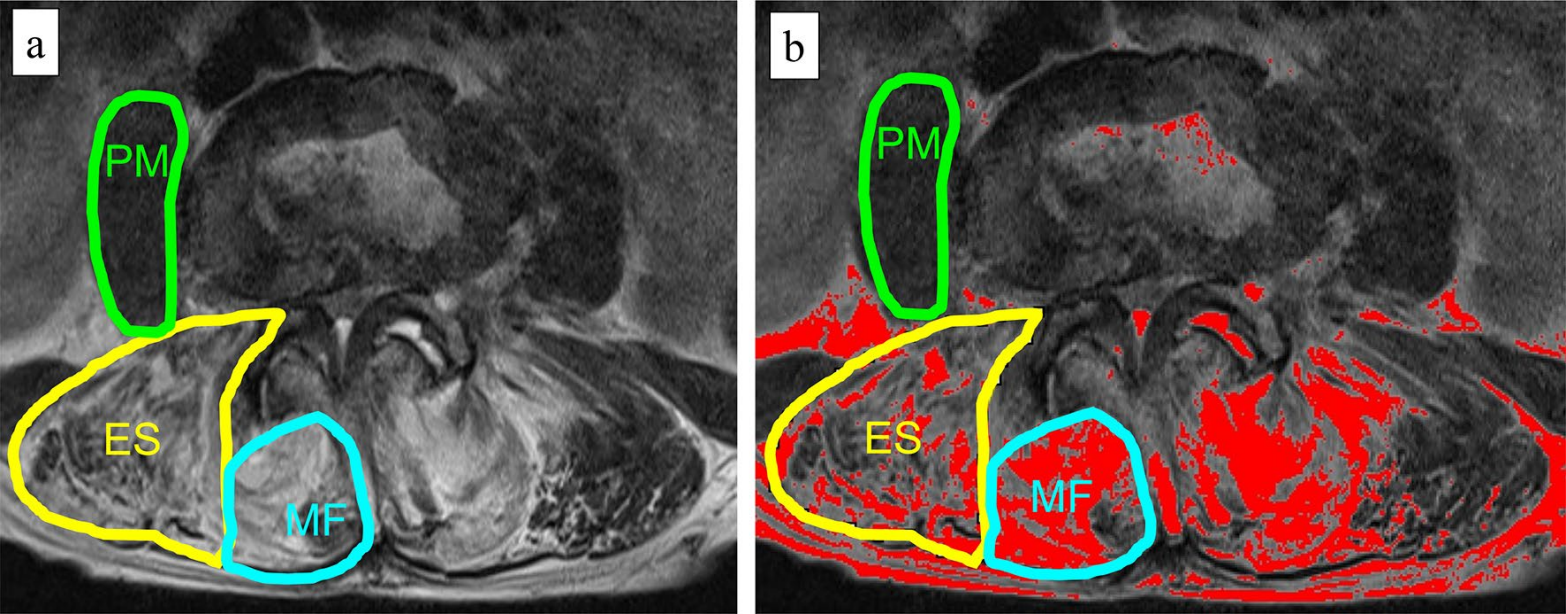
Calculation of the fat infiltration rate defined as the proportion of the area of the intramuscular fat tissue to the area of the regions of interest (ROI) of lumbar paravertebral muscles. (a) Manually traced regions of interest (ROI) around the erector spinae muscles, multifidus muscles and psoas major muscle at the L4-5 disc level on axial T2 weighted MRI images. (b) The intramuscular red area showing the fat signal area in the lumbar back muscles by using Image J software (National Institutes of Health, Bethesda, MD, USA). ES, erector spinae muscles; MF, multifidus muscles.
